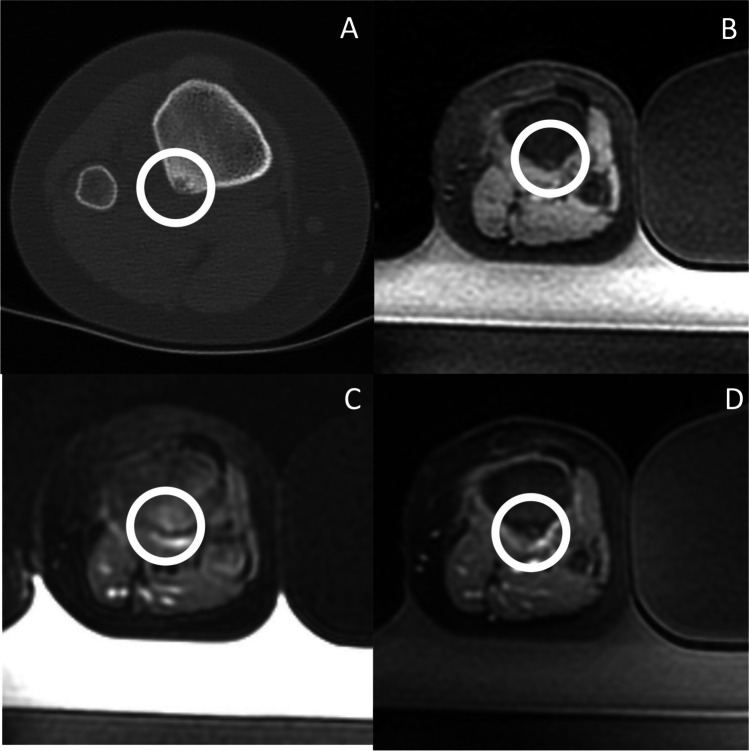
Osteoid osteoma. A Axial CT image in a patient who developed recurrent night pain relieved with NSAIDS approximately 10 months after initially successful CTgRFA for osteoid osteoma. The circle demonstrates an area of persistent subcortical lucency consistent with recurrent or residual nidus. B Pre-treatment axial T1-weighted FSPGR image demonstrates slight hyperintensity at the location of the nidus, highlighted by the circle. C Pre-treatment axial T2 FS image demonstrates subtle bone marrow edema pattern and overlying soft tissue edema at the site of the nidus, highlighted by the circle. D Post-treatment axial T1-weighted FSPGR post-contrast image demonstrates mild hypoenhancement at the treated nidus and mild hyperenhancement of the overlying soft tissues, highlighted by the circle. The patient’s pain completely resolved the day following the treatment
